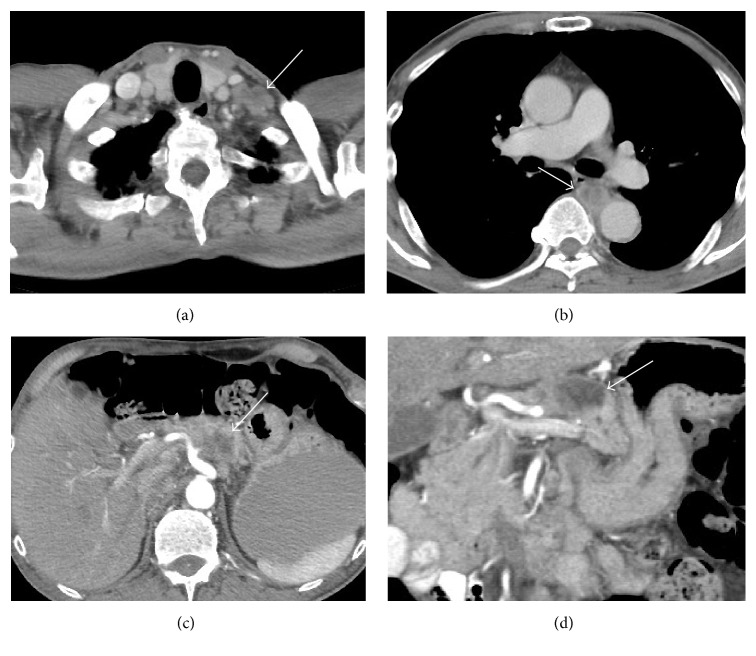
Computed tomography. (a) A conglomerate of lymph nodes in the left supraclavicular fossa measuring 3 cm (arrow). (b) A 2 cm left mid lung mass posterior to the left main stem bronchus (arrow). (c and d) A 3.8 cm hypodense mass in the pancreatic body with associated ill-defined soft tissue inseparable from the distal celiac axis and its branches (arrow).
